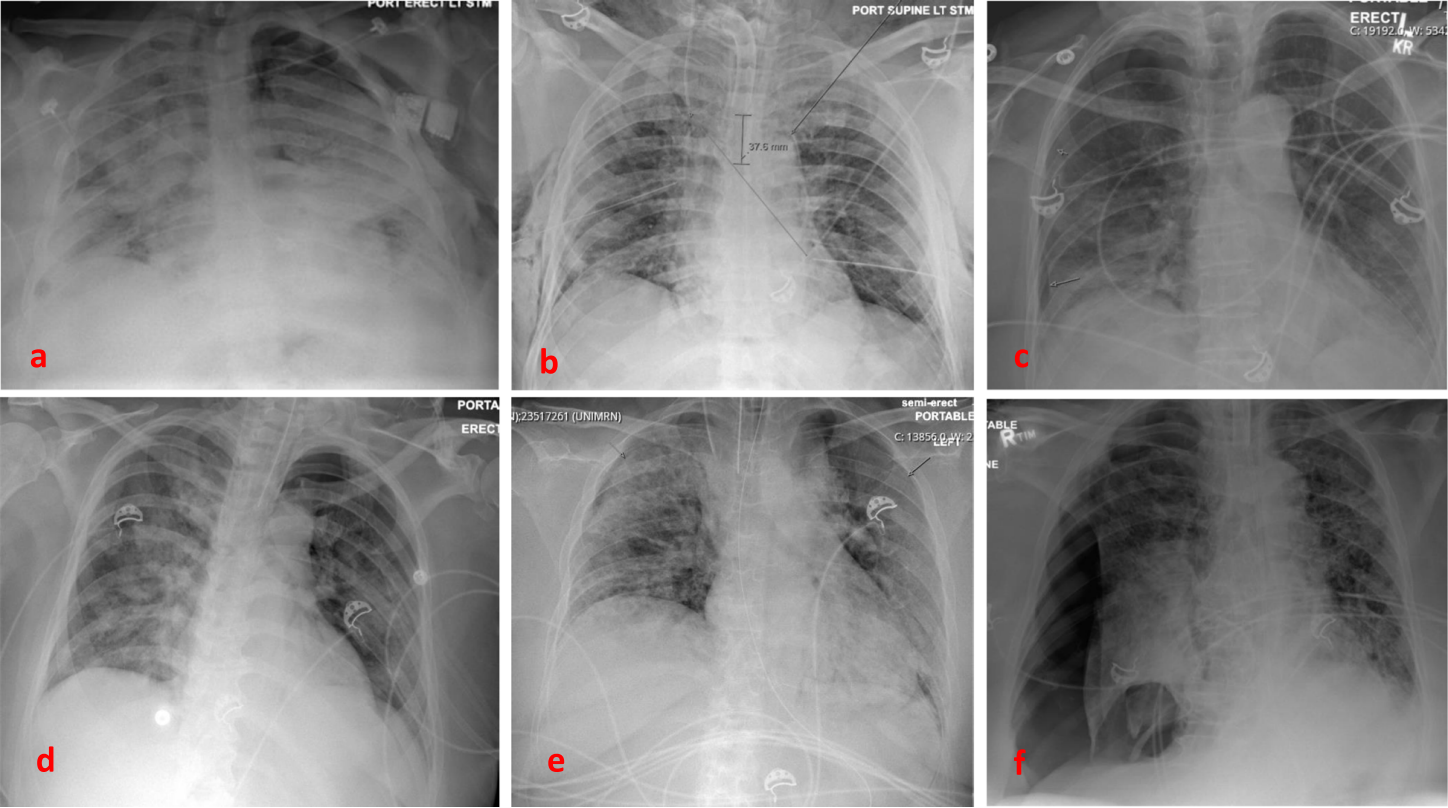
Chest radiograph for each patient revealing the pneumothorax. From top left: a Case-1 Large, left. b Case − 2 after pneumothoraces developed and chest tubes were placed. Residual right sided subcutaneous emphysema. c Case − 3. Moderate, right. d Case-4. Small, left apical e Case-5. Small, bilateral f Case- 6. Large, right
